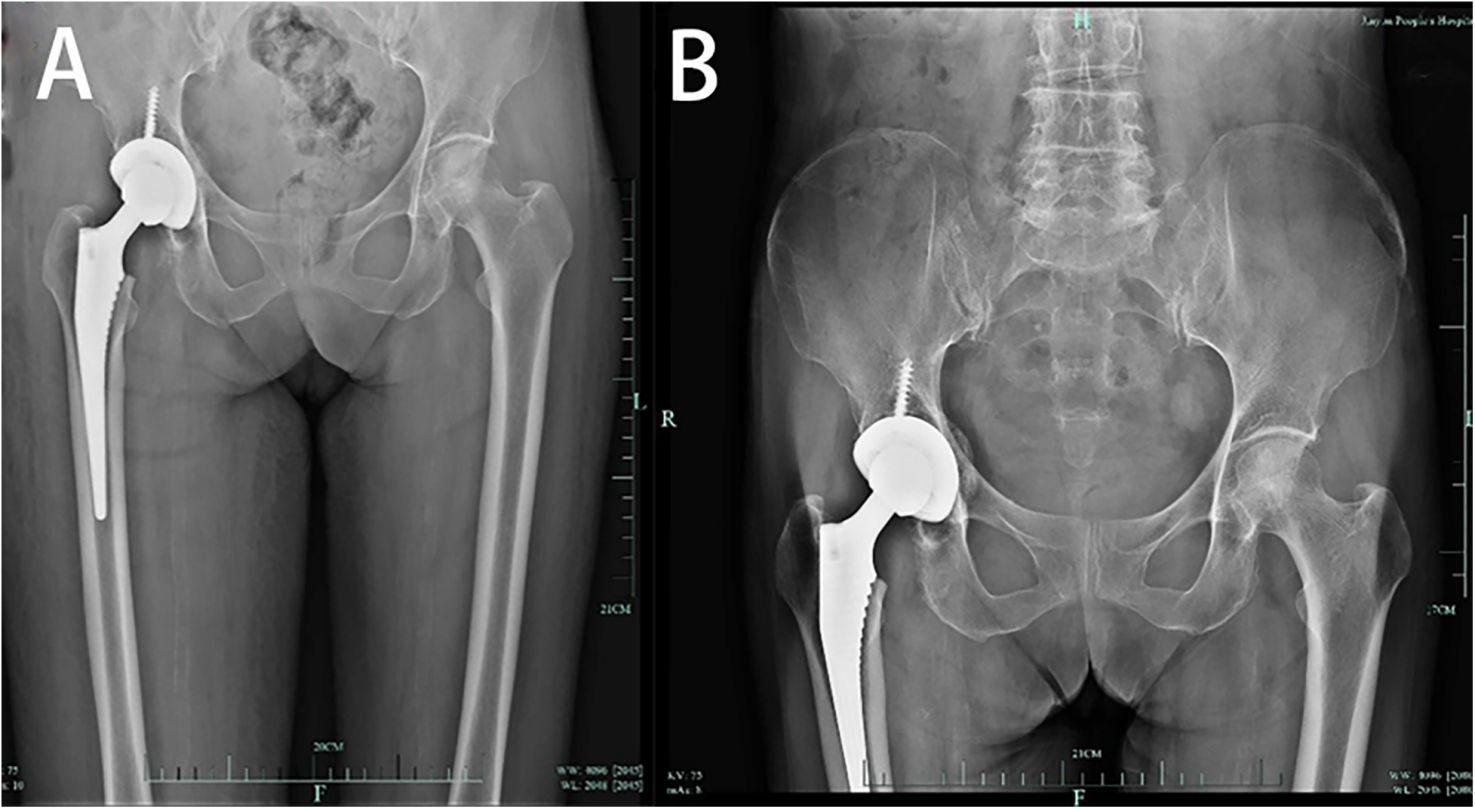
(A) X-ray reexamination after operation; (B) X-ray was reexamined after 3 months.
